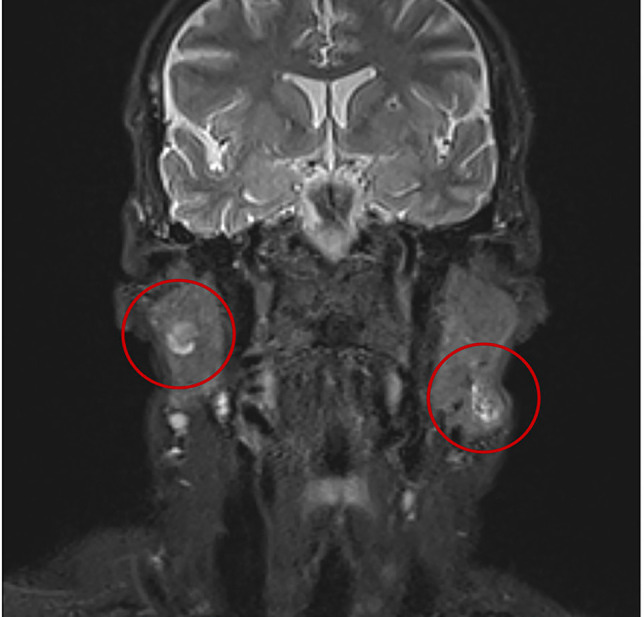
Initial magnetic resonance imaging (MRI) in T2-weighted STIR 3D sequence showing multiple bilateral parotid nodules, appearing as hyperintense lesions within both parotid glands.
